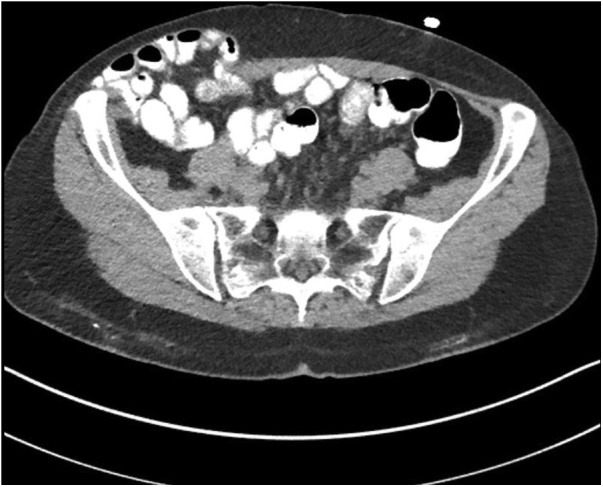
CT cut through the hernia in the right lower quadrant. By CT, it measured 8 cm in diameter. The skin and some subcutaneous tissue were intact over the hernia defect.
